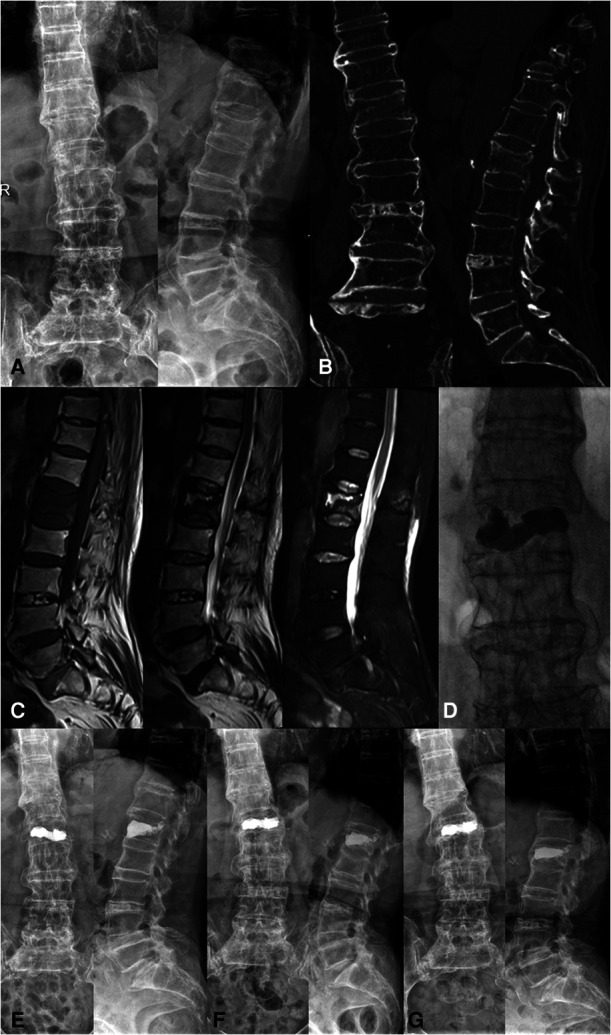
(A) X-rays before surgery. (B) CT before surgery. Wave-shaped ossification was observed at the anterolateral side of the spine. The L1 fracture was also shown. (C) MRI including T1, T2, and STIR before PKP. (D) X-rays taken by C-arm fluoroscopy during the operation. Bone cement was injected into the L1 vertebra. (E) X-rays at 1 day after surgery. (F) X-rays at 2 months after surgery. (G) X-rays at 6 months after surgery.
